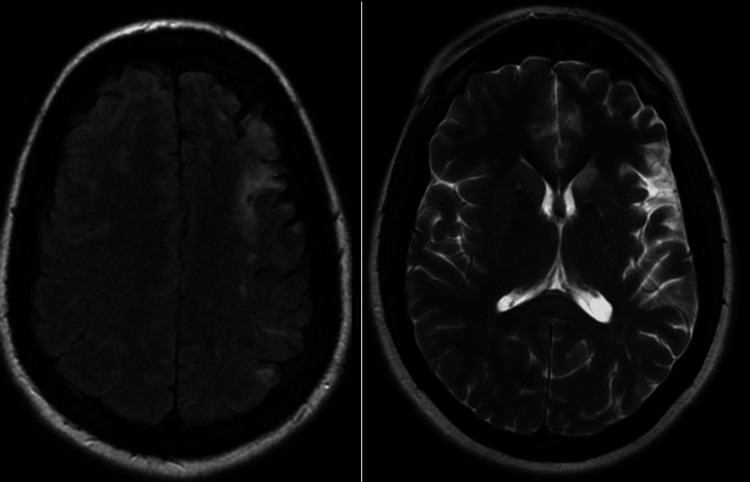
T2 FLAIR demonstrating a large ischemic lesion in the left MCA and ACA territories (left). T2 PROPELLER redemonstrating the left frontoparietal and the left caudate head lesions.
